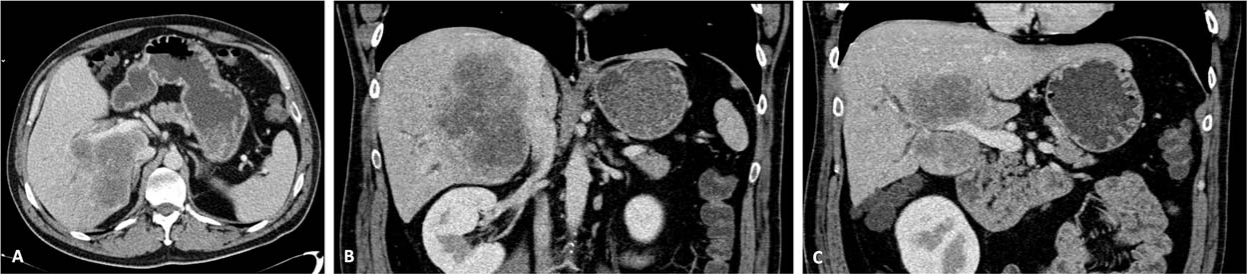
CT scan of the intrahepatic cholangiocarcinoma before chemotherapy. (A) Axial CT scan of the intrahepatic cholangiocarcinoma centred in liver segments 5, 7, and 8, with extension to segment 1 and invasion of the PV and IVC; (B) coronal CT scan view of the vascular relation between the cholangiocarcinoma and the IVC; and (C) vascular invasion of the PV.
